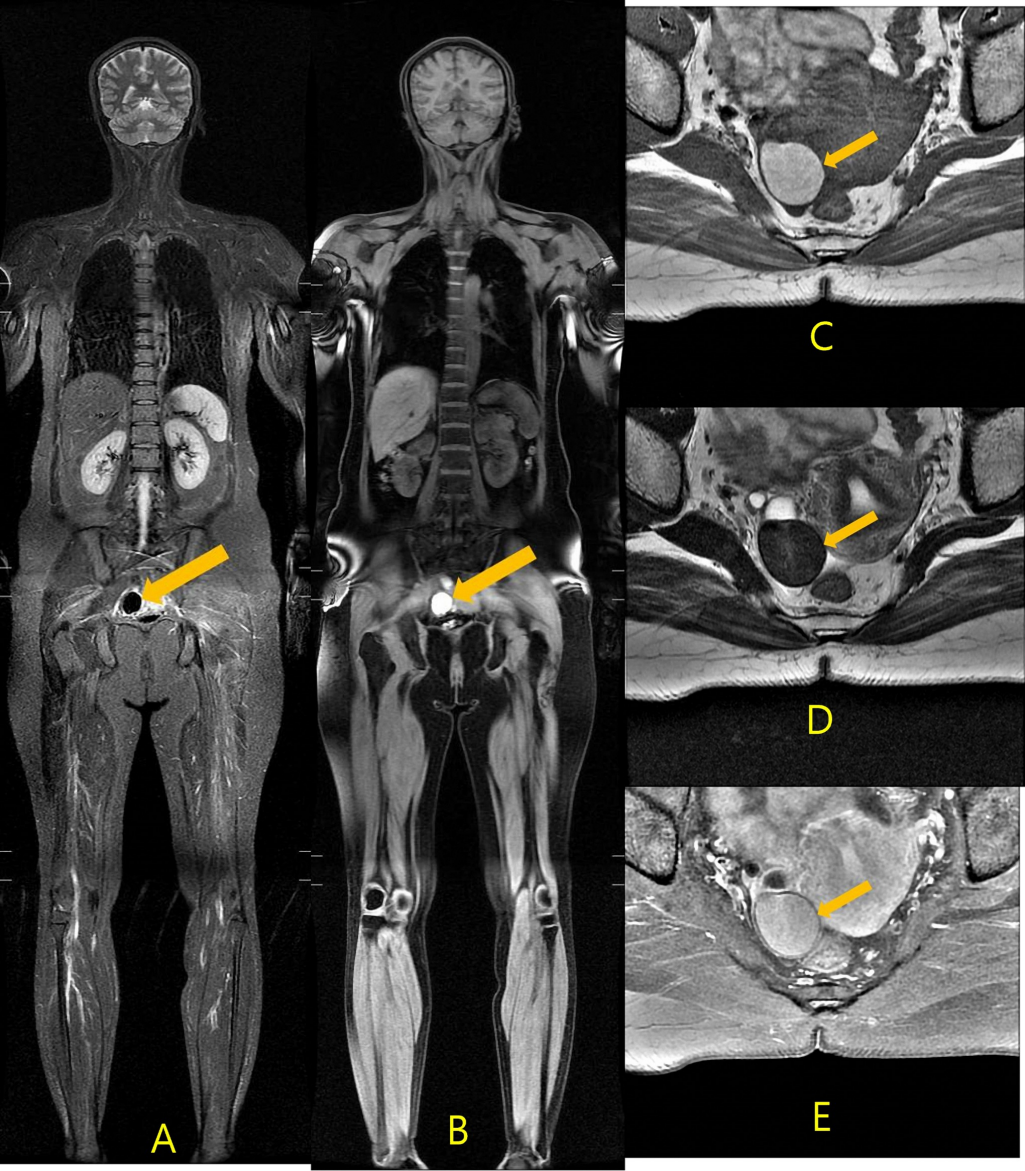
A 43-year-old woman who underwent a routine health examination. Coronal T_2_-weighted STIR image (TR/TE, 6000, 2800/35, A) and coronal T_1_ fat suppression SPGR (TR/TE, 7.6, 6.3/3.6, B) images revealed a well-defined, 3-cm hemorrhagic mass in the pelvic cavity (arrow). The initial radiologic diagnosis was endometrioma. Radiologist reviewers classified this lesion as category II. The follow-up axial MRI, including T_1_-weighted MR image (TR/TE, 600/9, C), T_2_-weighted MR image (TR/TE, 3700/100, D) and fat-saturated enhanced T_1_-weighted MR image (TR/TE, 800/10, E), revealed a 3-cm well-defined oval shaped mass at the right adnexa suggestive of an endometrial cyst (arrow).
